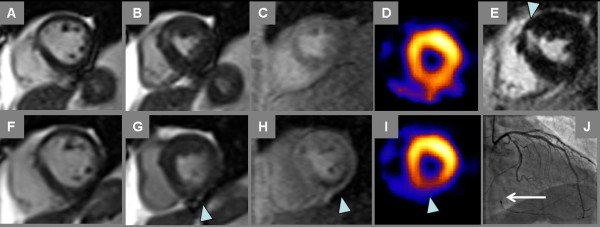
Ischemia by Treadmill Stress CMR and SPECT. Rest and stress CMR and SPECT images in the same patient of Fig. 3 both demonstrate myocardial ischemia, with corresponding obstructive coronary artery disease by angiography. Resting diastolic (A) and systolic (B) cine frames vs. comparable post-exercise cine frames (F, G) show stress-induced inferior wall contractile dysfunction (G, arrowhead). Inferior ischemia is also demonstrated by CMR perfusion imaging (C-rest perfusion vs. H-stress perfusion, arrowhead). Prior MI in the anteroseptum can be seen on late post-gadolinium imaging (E); note some fatty replacement in the infarct region evident as bright intramyocardial signal on noncontrast gradient echo cine frame in panel B. Rest Tc-99 m perfusion SPECT (D) suggests normal perfusion, though somewhat obscured by adjacent bowel uptake; stress Tc-99m perfusion SPECT shows inferior wall defect (I, arrowhead). The patient went on to invasive angiography that showed an occluded right coronary artery (J, arrow) with some left-to-right collateral flow.
